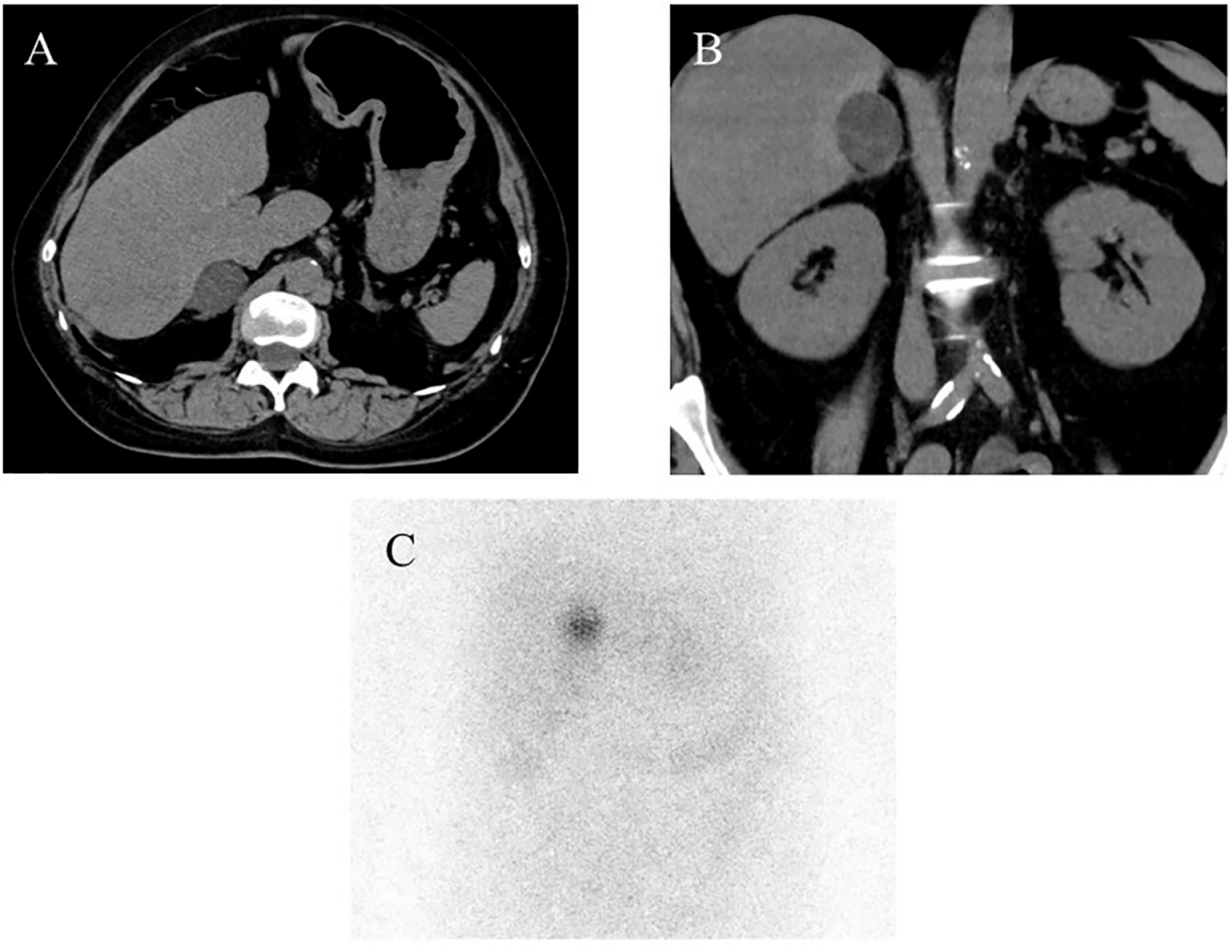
Imaging analysis. (A, B) Non-enhanced computed tomography (CT) demonstrated the presence of a right adrenal tumor, (C) 131I-adosterol adrenal scintigraphy revealed increased uptake of adosterol in the right and suppressed one in the left adrenal glands, respectively.
